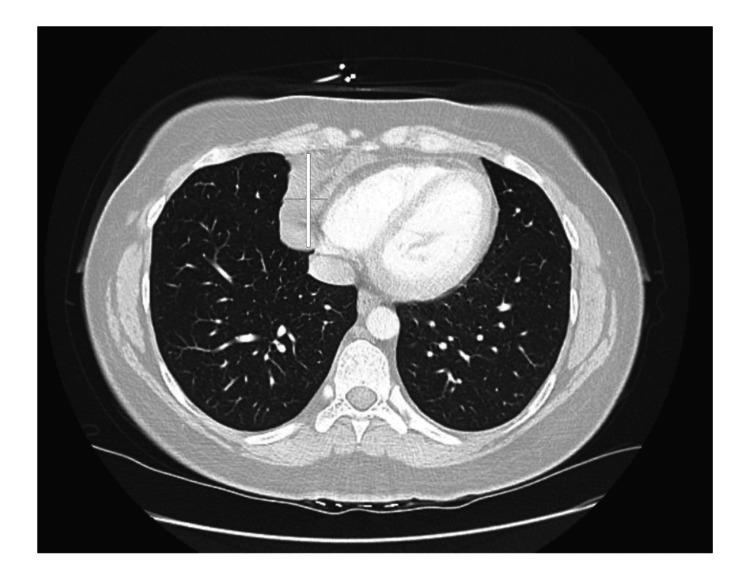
Computed Tomography Angiography (CTA) chest axial view. An axial CTA view of a pericardial cyst fluid in the lower anterior mediastinum indicated via the vertical and horizontal lines. Measurement about 6.0 x 5.4 x 6.4cm.
